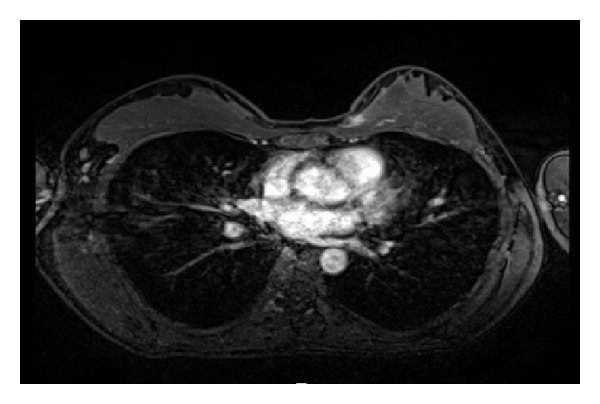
Magnetic resonance imaging (T1-weighted image, fat suppression) showed enhancement of the lesion.
